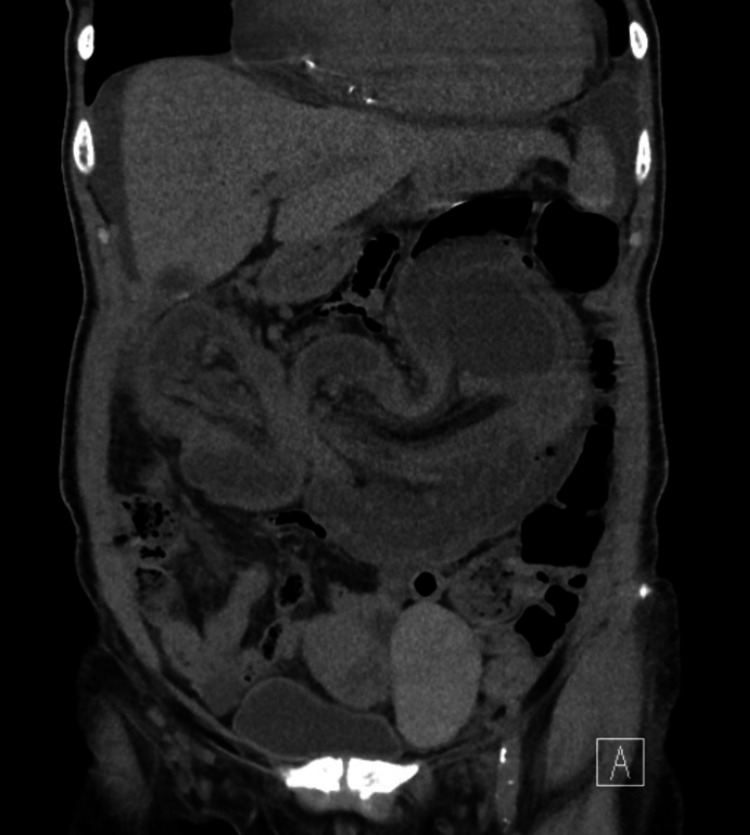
Coronal slice of the CT of the abdomen/pelvis demonstrating a large colonic intussusception in the mid-abdomen with extensive wall thickening and edema.
